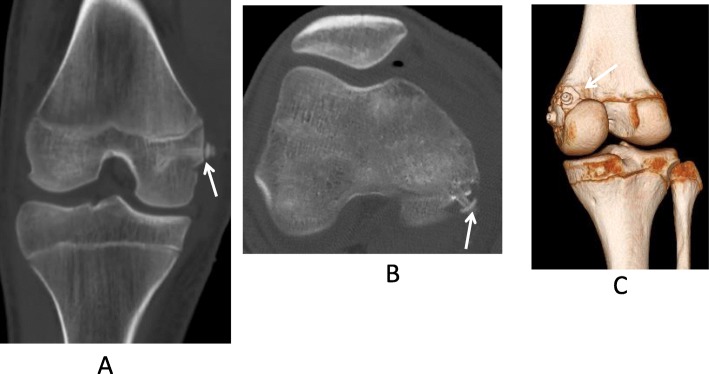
a and b and c Computed tomography of the injured knee after surgery. An avulsion fracture of the medial collateral ligament was reduced and fixed with an absorbable screw (*arrow*, a). An avulsion fracture of the medial head of the gastrocnemius muscle was reduced and fixed with an absorbable screw in the axial plane (*arrow*, b), and in three-dimensional computed tomography (*arrow*, c)
